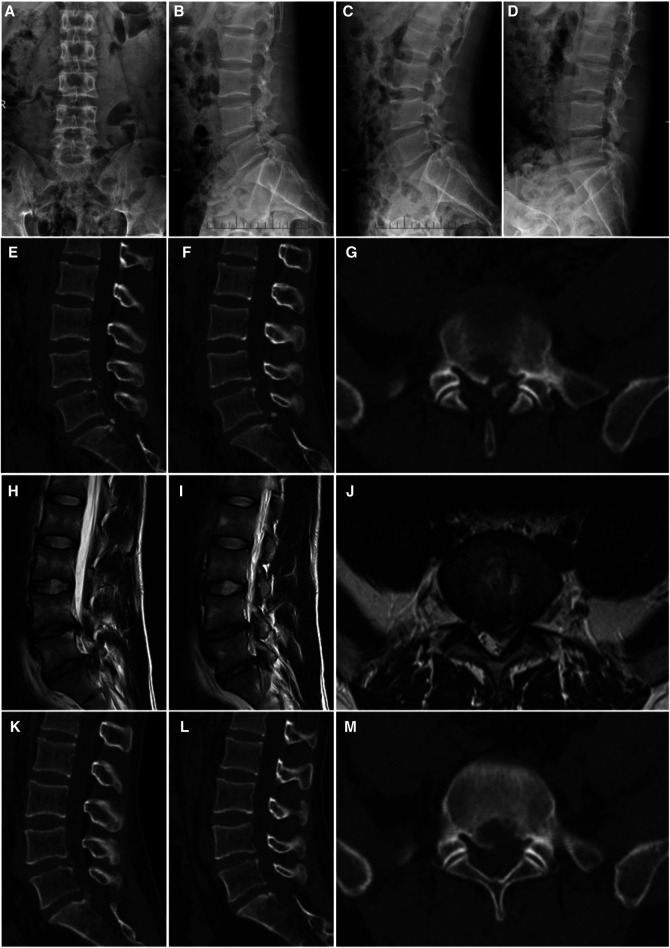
A 26-year-old man with LDH linked with PRAS was treated with PETD; the main symptoms were low back pain with radiating pain in the left lower limb. (A,B) anterior and lateral X-ray images of the lumbar, (C,D) dynamic X-ray images of the lumbar, (E–G) CT scan images of the lower lumbar spine: the detached bone fragment is shown to protrude into the posterior margin of the vertebral body, (H–J) MRI of the lumbar spine: the imaging findings were consistent with that of the CT scans, and some of the discs are shown to protrude laterally, (K–M) the CT scan images show that the bone fragment pressing the nerve on the patient's left side has been removed.
